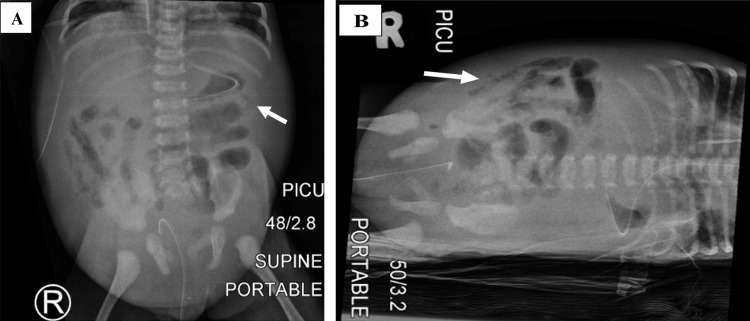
Abdominal radiographs: (A) supine and (B) left lateral decubitus radiographs showed radiologic features of pneumatosis intestinalis (white arrow). White arrow: pneumatosis intestinalis
